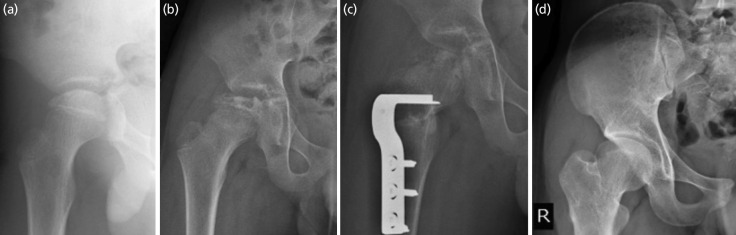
The series of radiographs belongs to patient no.4. (a) The presentation at the age of 10 showed ischemic phase. (b) Early fragmentation phase with Herring B at age of 11. (c) The immediate post-surgery showed good containment. (d) The hip at 21 years old showed good outcome with Stulberg II.
